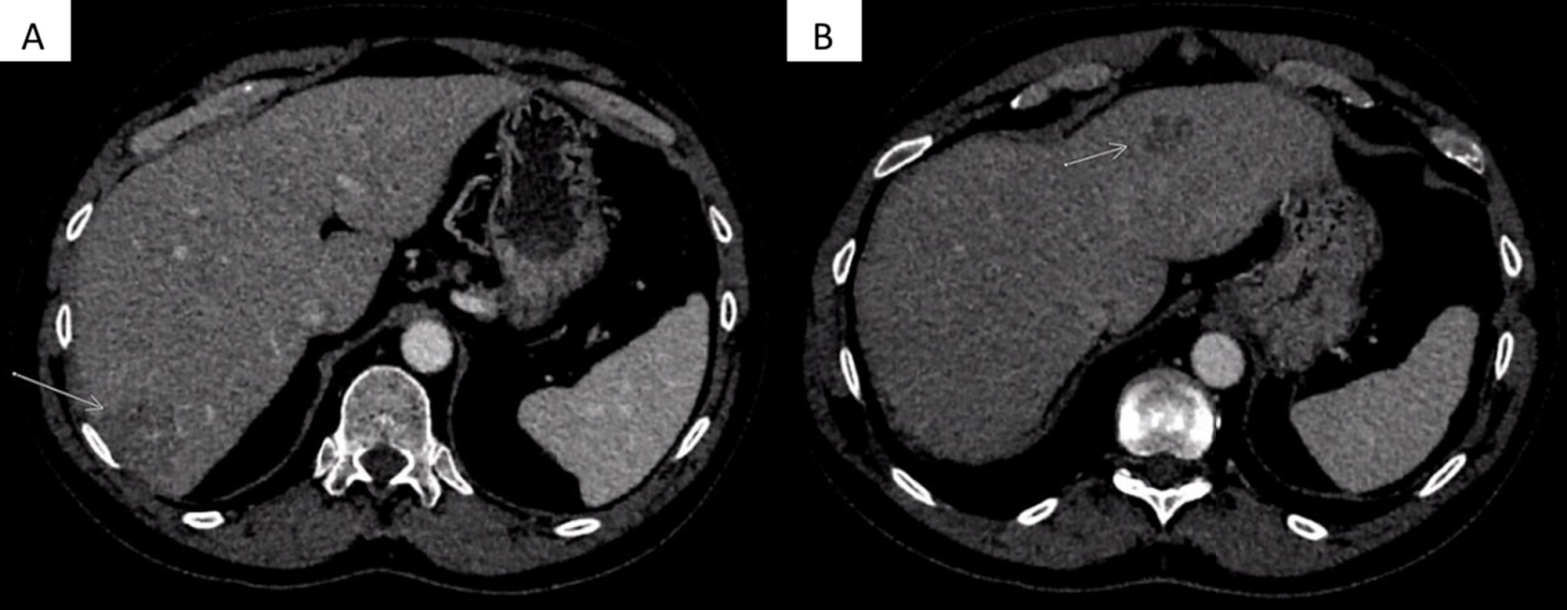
Interim CT images while on adjuvant chemotherapy Axial contrast-enhanced CT images through the liver demonstrate a discrete appearance of the low attenuating mass in the right hepatic lobe (arrow in A) seen on prior MRI. In addition, there is a new, smaller mass in the left hepatic lobe (arrow in B) that was not seen on prior imaging.
